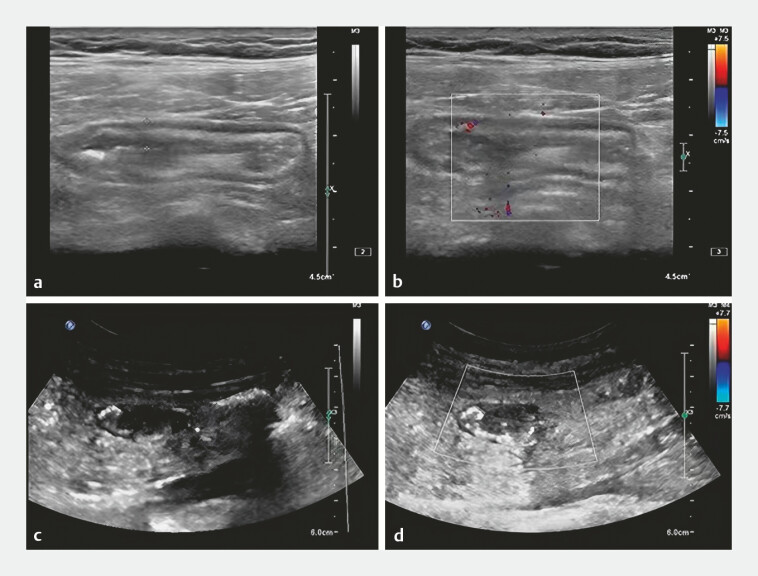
Intestinal ultrasonography follow-up showing improvement in bowel wall thickness from 7.0 mm to 5.6 mm and Limberg grade from 1 to 0.
